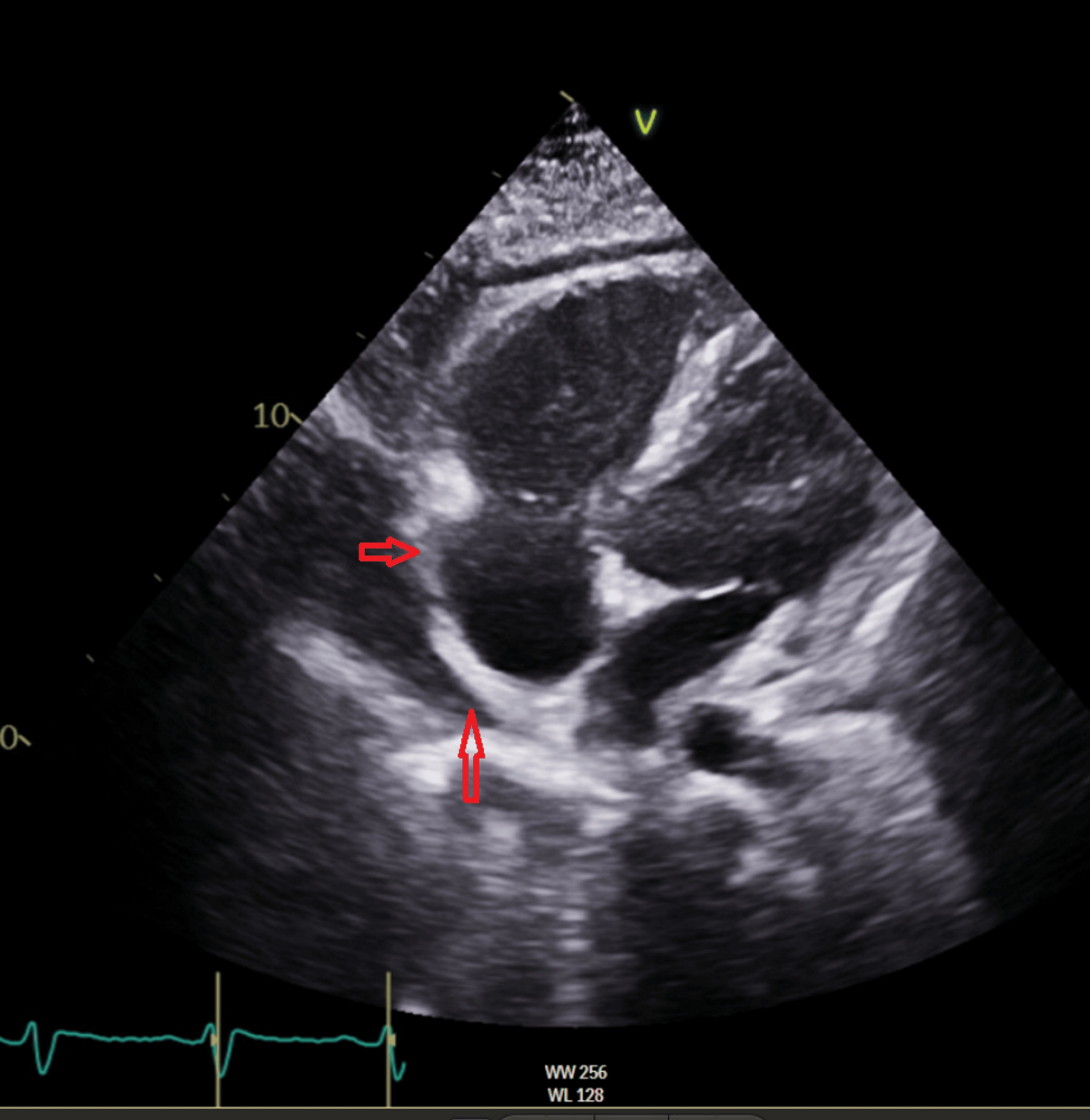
Transthoracic echocardiogram image showing a small to moderate pericardial effusion.
